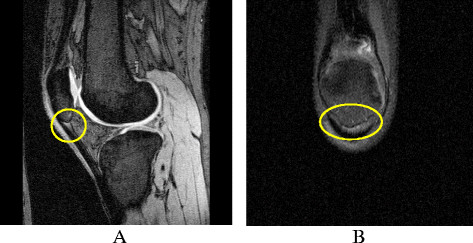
Patellar tendinopathy on MRI on A. T1-weighted fat-saturated sagittal MRI; and B. T2-weighted fat-saturated coronal MRI.
